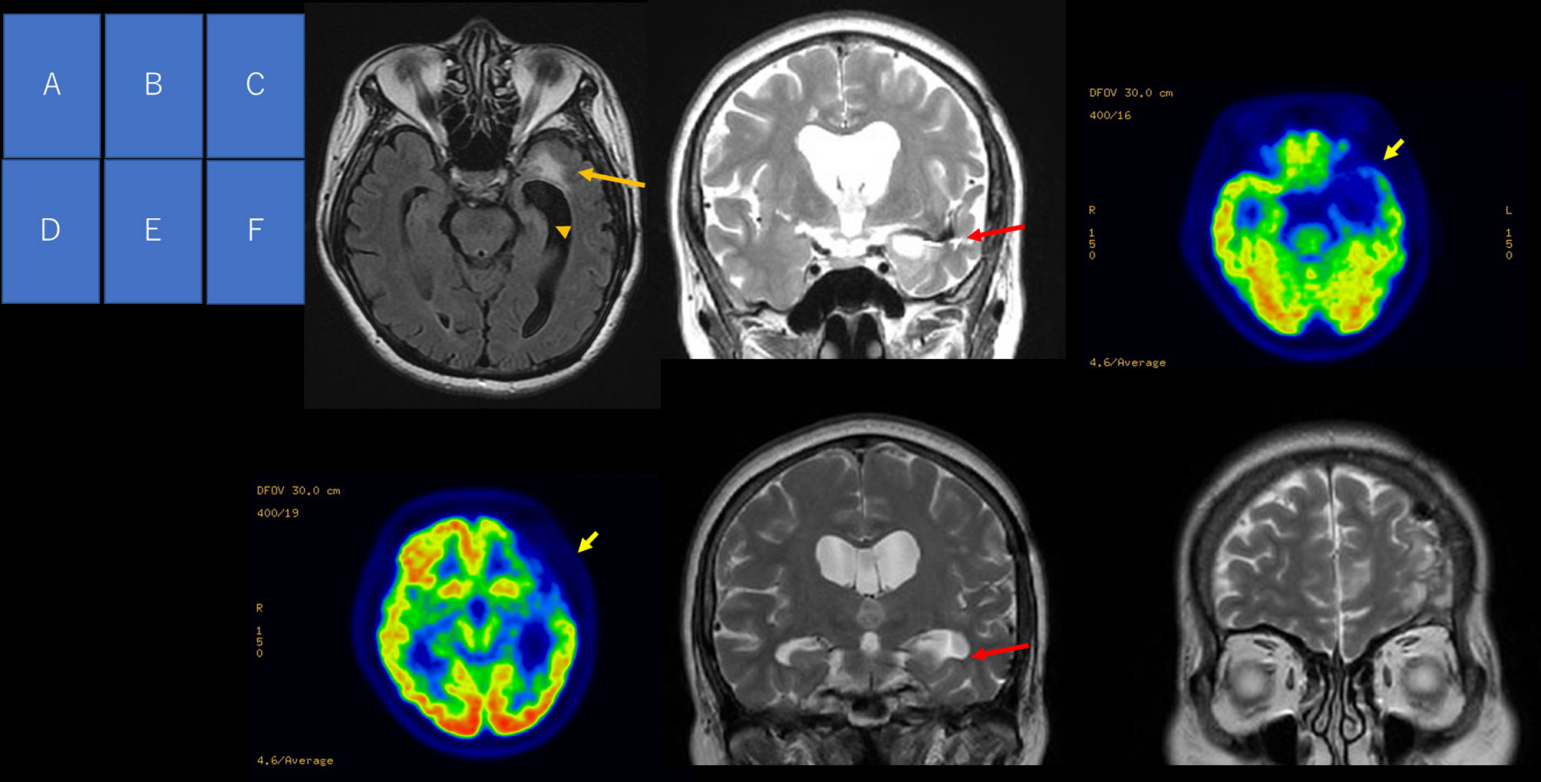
Pre-operative and post-operative neuroimaging. MRI FLAIR axial image (A) showing a hyperintense signal at the left temporal pole (arrow) and hippocampal atrophy (arrowhead). T2-weighted coronal image (B) showing a hypointense signal along the lower insula cortex and the roof of the inferior horn. Positron emission tomography (C,D) showing reduced glucose uptake in the left frontal and temporal lobes (arrow). T2-weighted coronal image (E) at the level of the hippocampal body showing hippocampal atrophy (arrow). T2-weighted coronal image at frontal region (F) showing a change in intensity.
